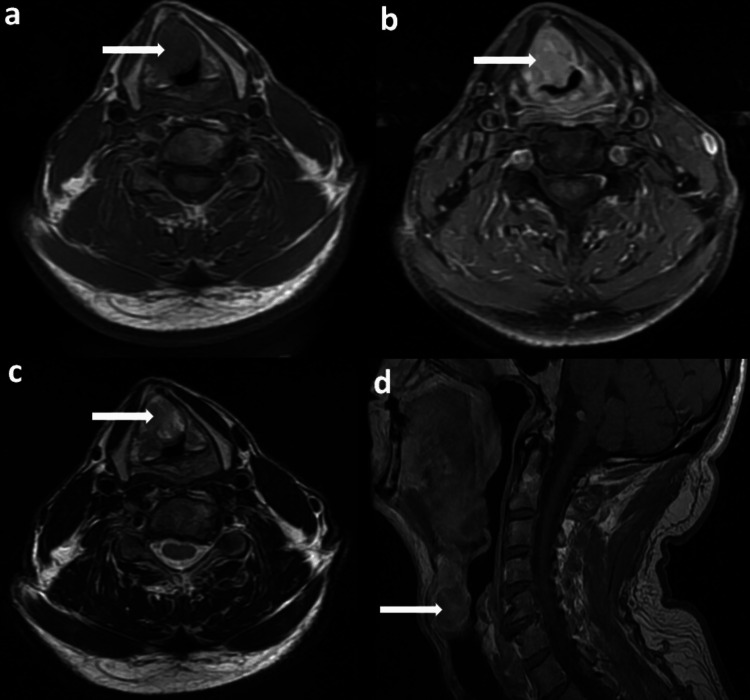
Magnetic resonance imaging of the neck after paramagnetic contrast enhancement. A laryngeal tumor (arrow) (30x20x22 mm) with no invasion of the surrounding structures. MRI (a) axial (T1W1 sequence), (b) axial (T1W1 sequence with fat-sat), (c) axial (T2W1 sequence), and (d) sagittal (T1W1 sequence).
